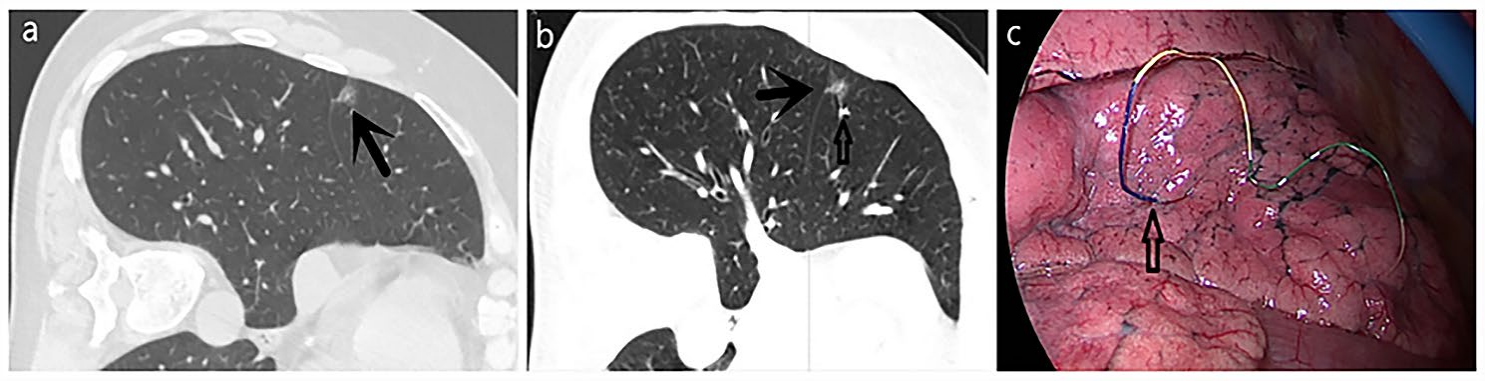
A 56-year-old female with a GGN in the right middle lobe who underwent CT-guided localization with a four-hook needle. (a) The lesion (black arrow) is observed on an axial CT image. (b) Postlocalization CT shows the relationship among the released anchor (white arrow), lesion (black arrow) and chest wall. (c) The end of the four-hook needle (white hollow arrow) is visualized during VATS.
